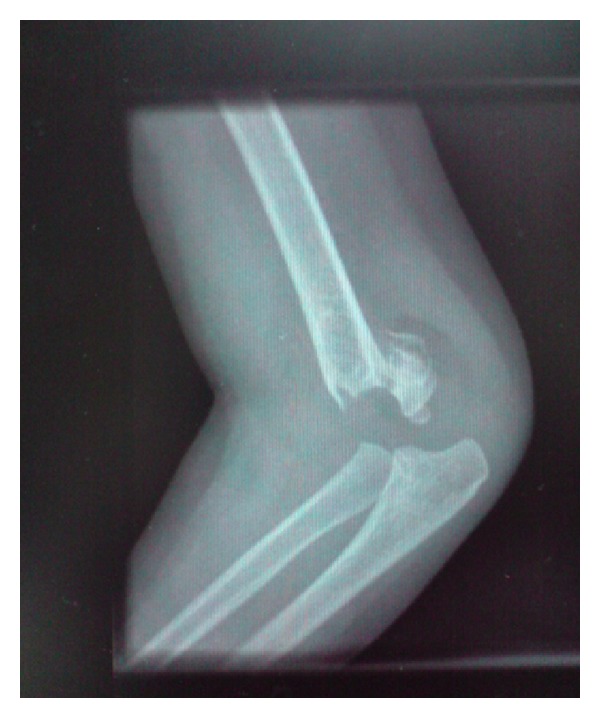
Preoperative lateral radiograph.
